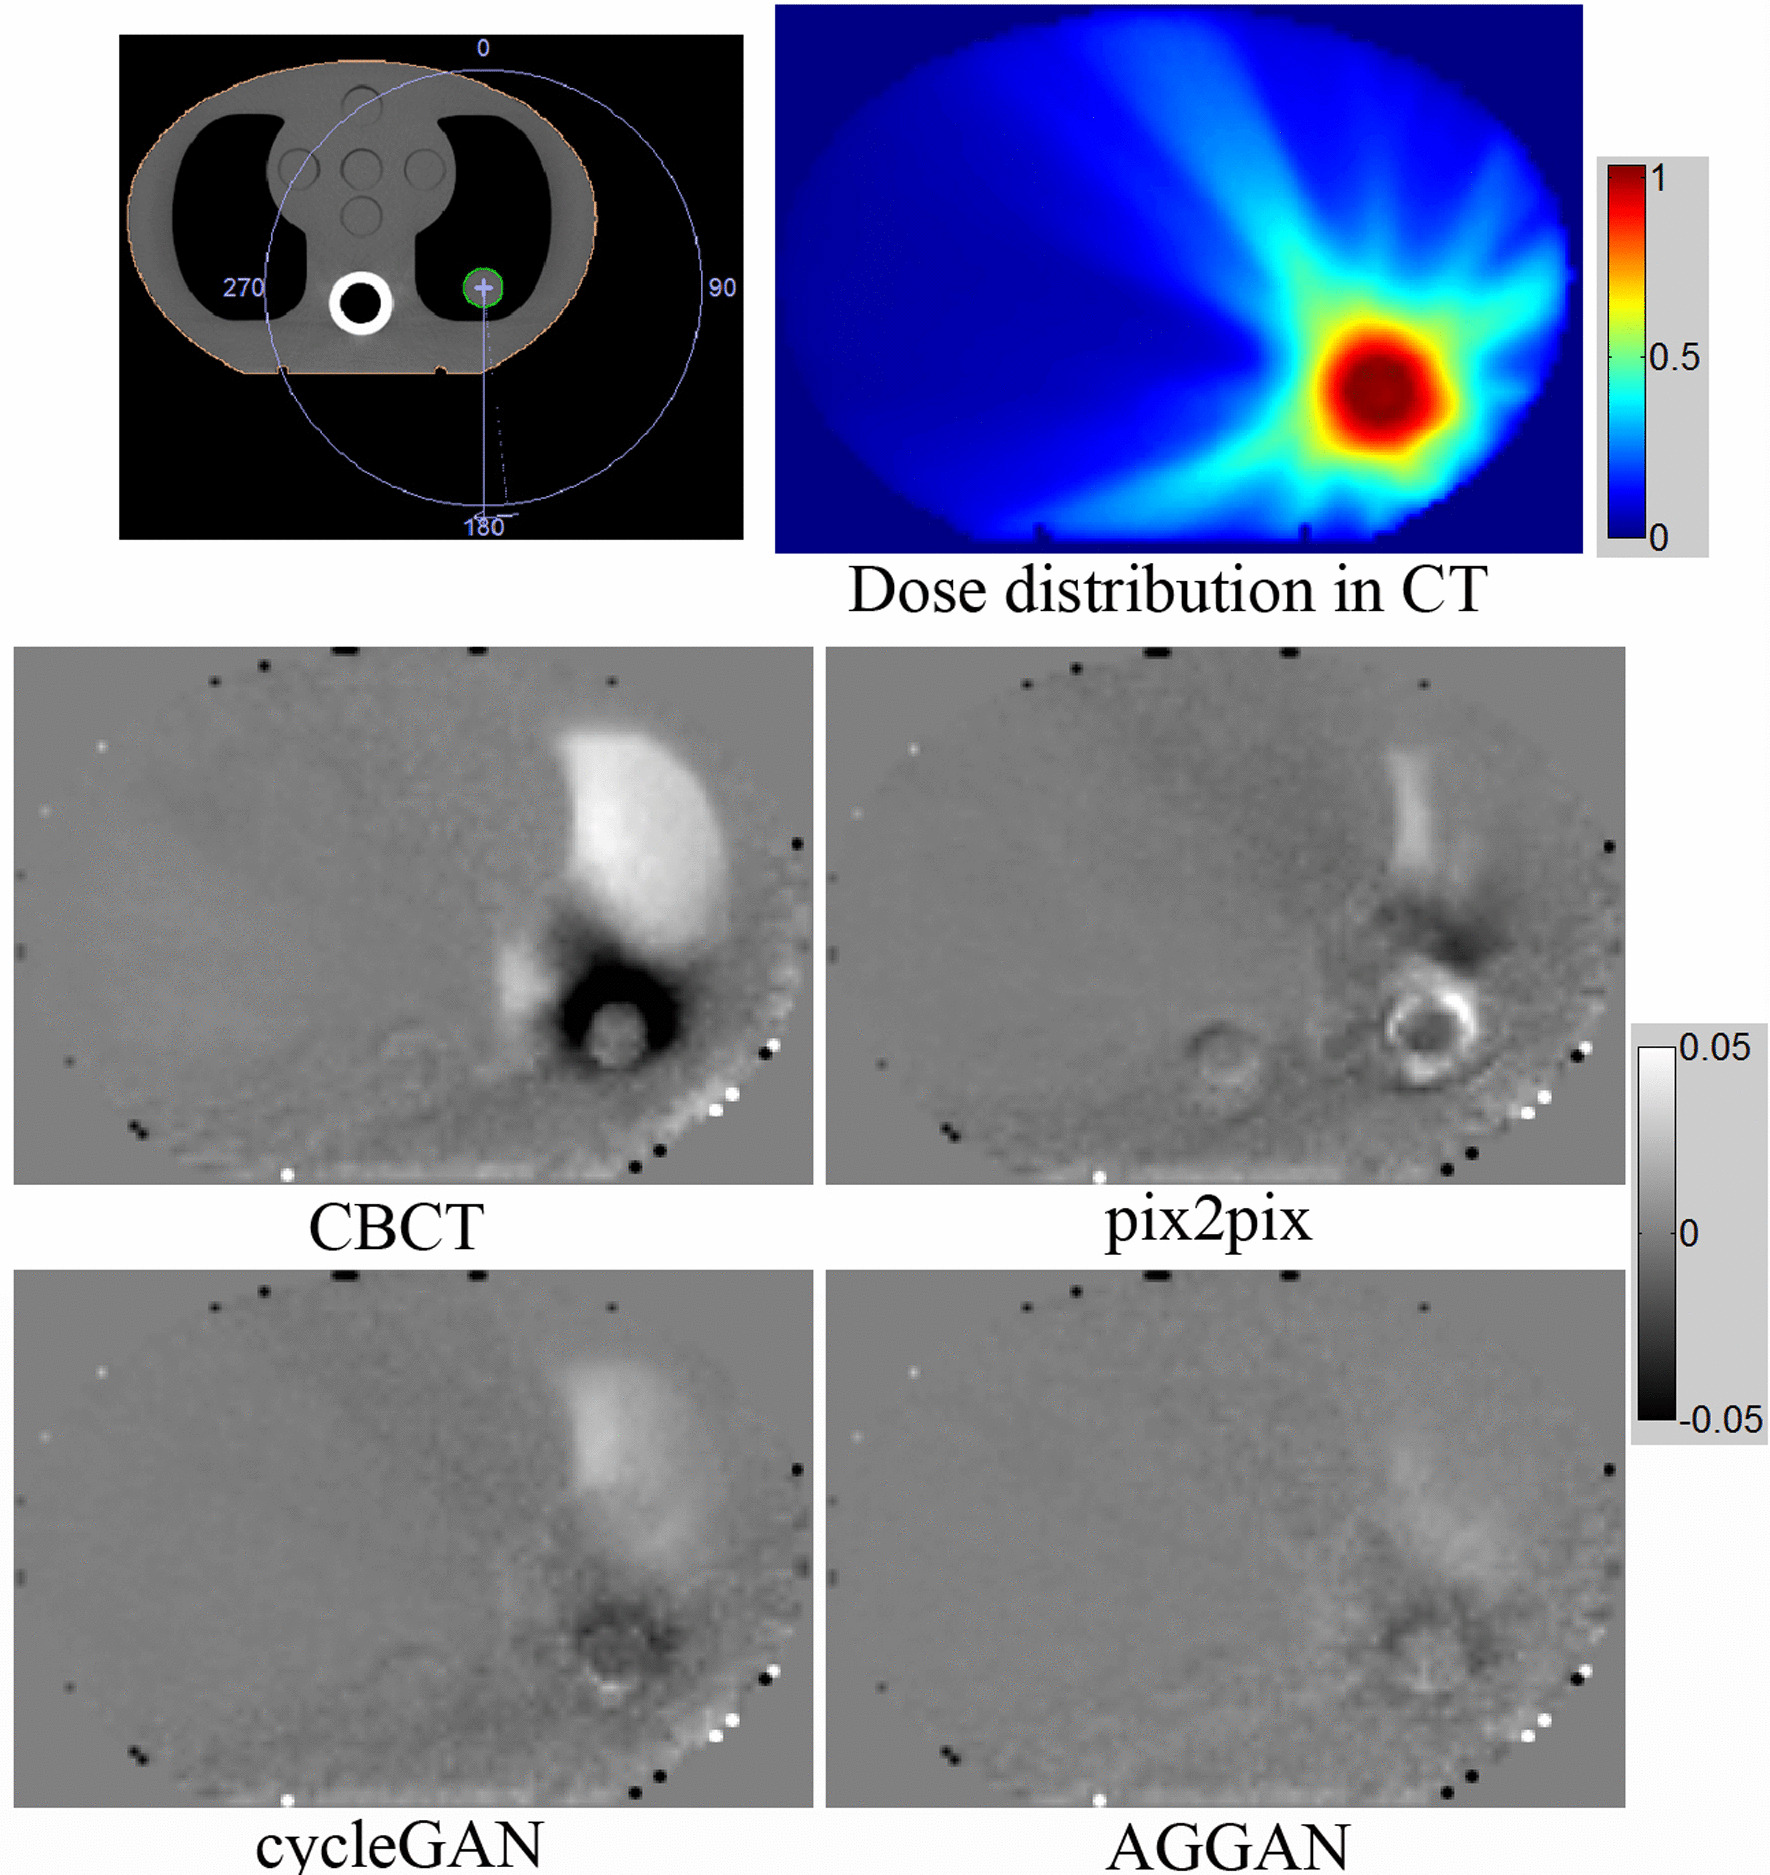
Dose distribution in CT images and distributions of relative dose differences in CBCT and sCT images genarated from pix2pix, cycleGAN and AGGAN
